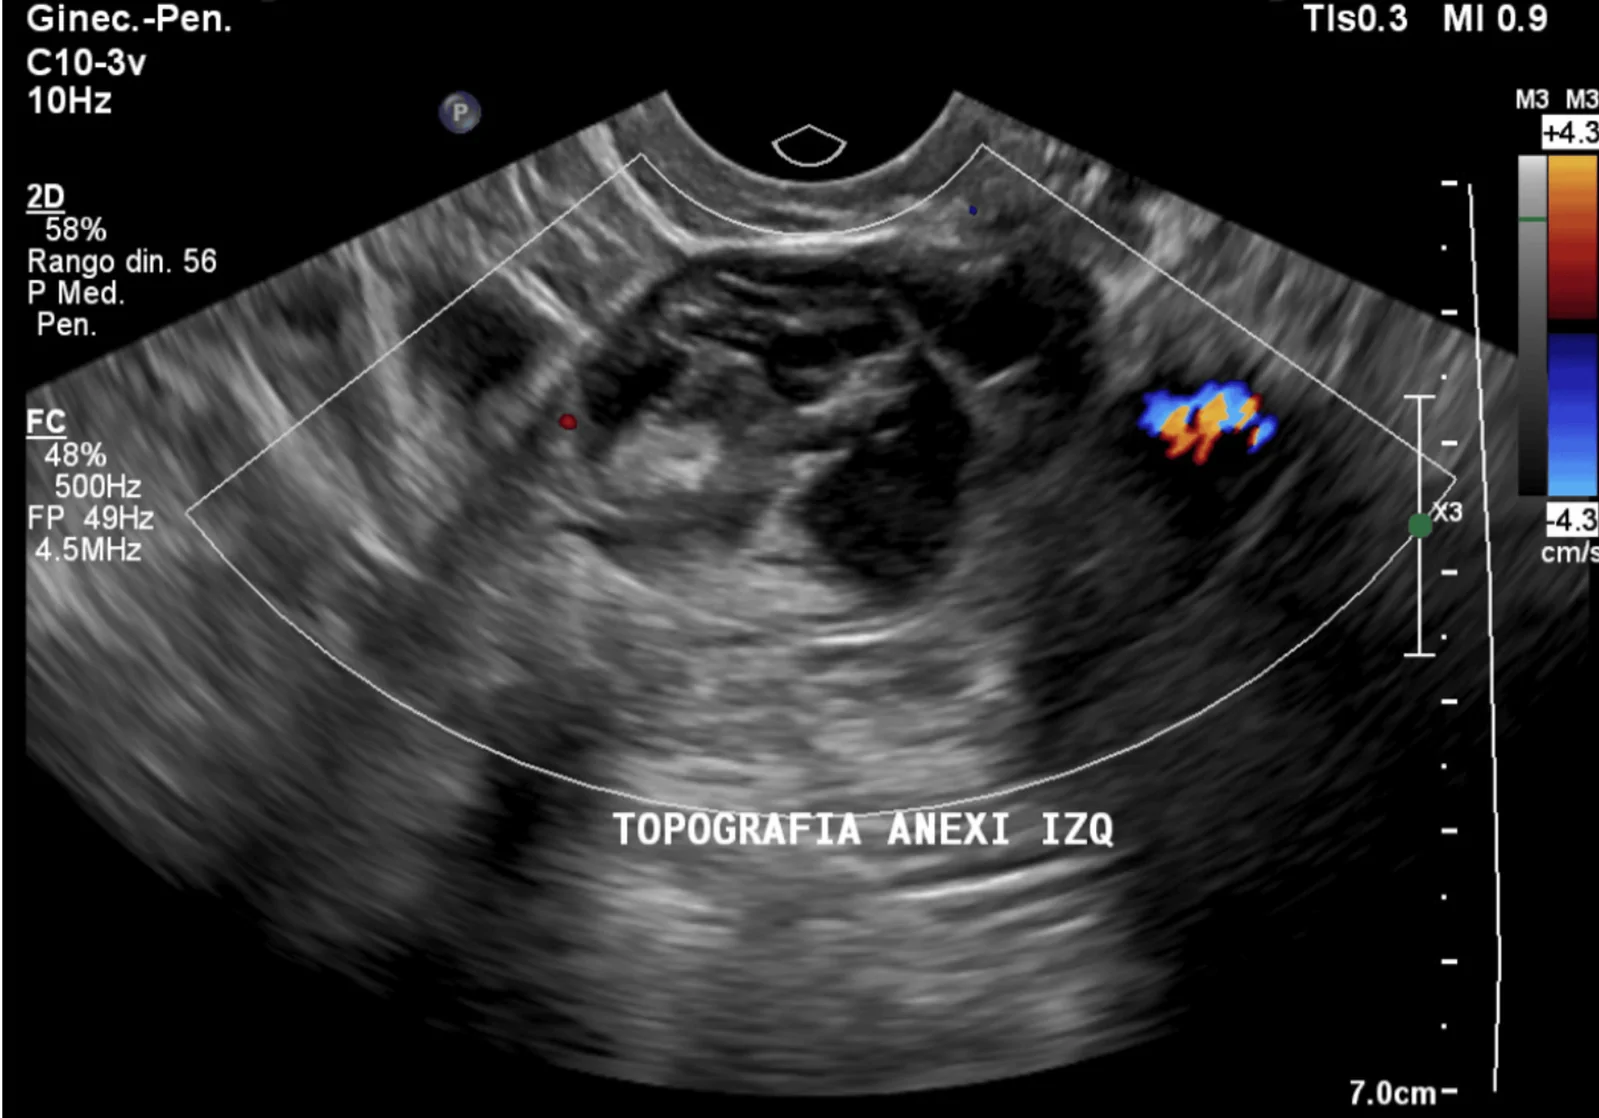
Follow-up endovaginal ultrasound demonstrating the interval growth of the left adnexal cystic mass Follow-up endovaginal ultrasound of the left adnexal region (TOPOGRAFIA ANEXI IZQ) showing a complex cystic mass with internal echoes and Doppler flow signal, with interval growth compared to prior imaging, prompting surgical intervention. The lesion measured 3.3 × 2.8 × 3.1 cm on this examination.
